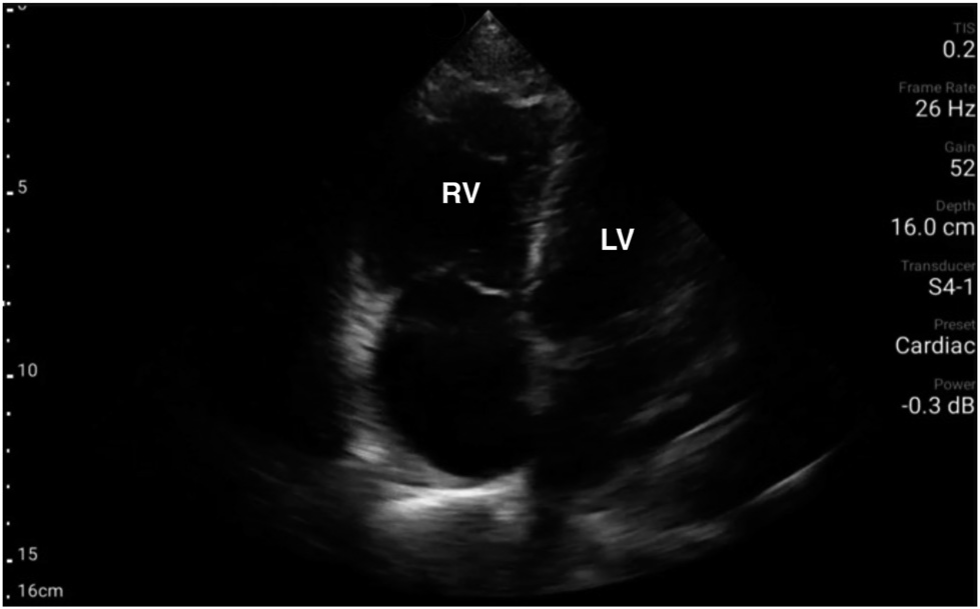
POCUS apical 4-chamber. Apical 4-chamber view of the heart at end-systole showing severely dilated and hypokinetic right ventricle (RV) and underfilled, hyperdynamic left ventricle (LV). See Video 1:
